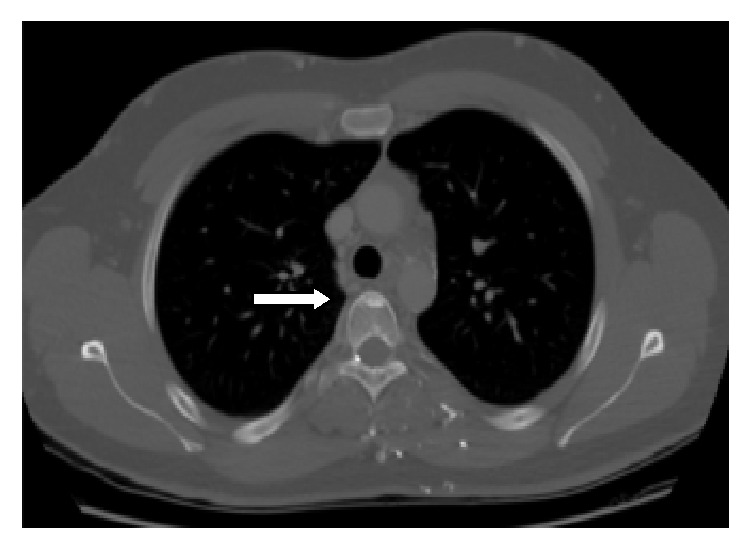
(Chest contrast enhanced CT)*: Axial bone window CT demonstrating a solitary osteoblastic lesion at T5 (white arrow).
